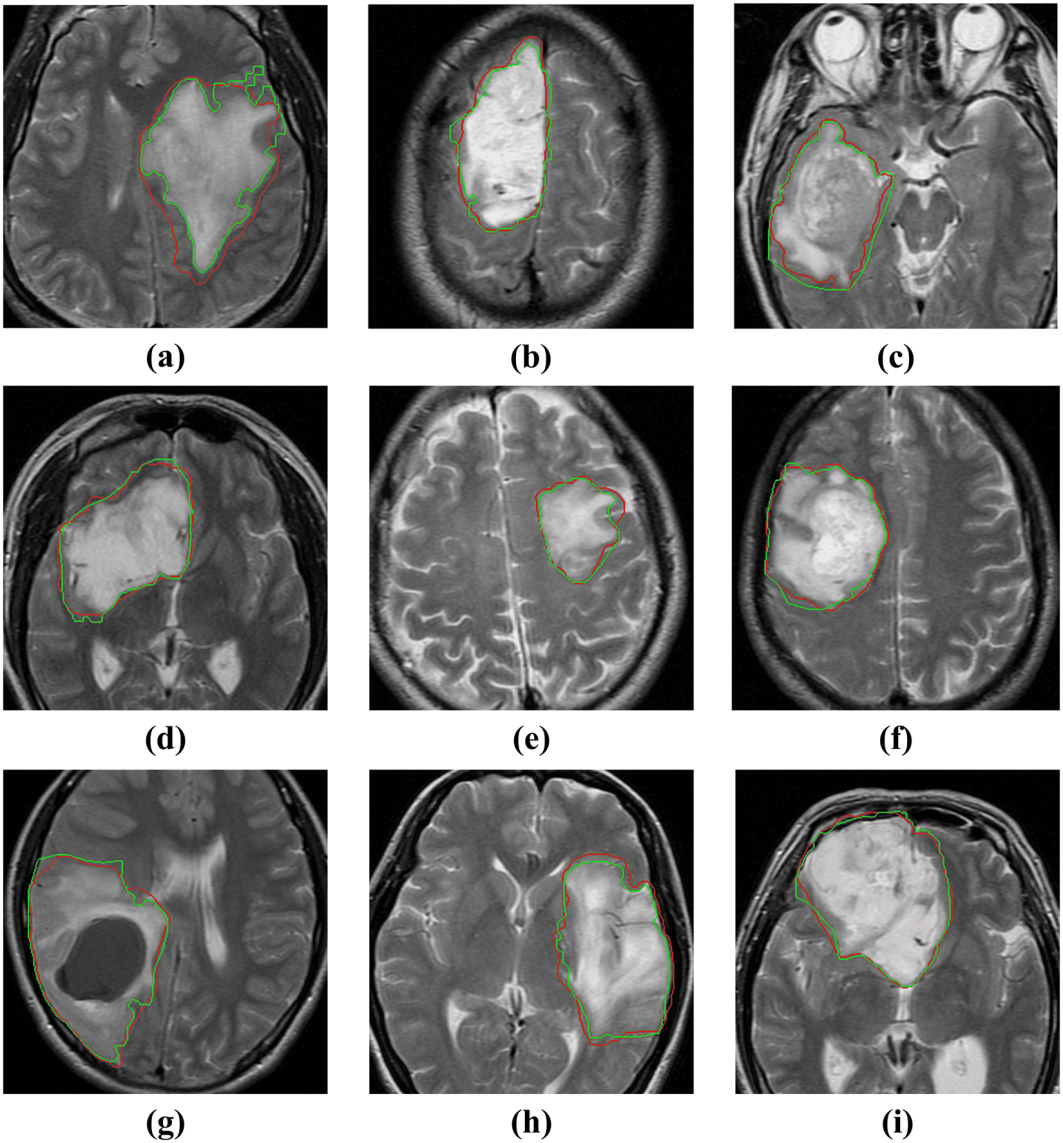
Comparison between manually delineated gross tumour volume (GTV) and automatically segmented GTV on axial slices of T2-weighted MRI images for 9 patients. (a–i) Represent patient No. 1~9 respectively.
